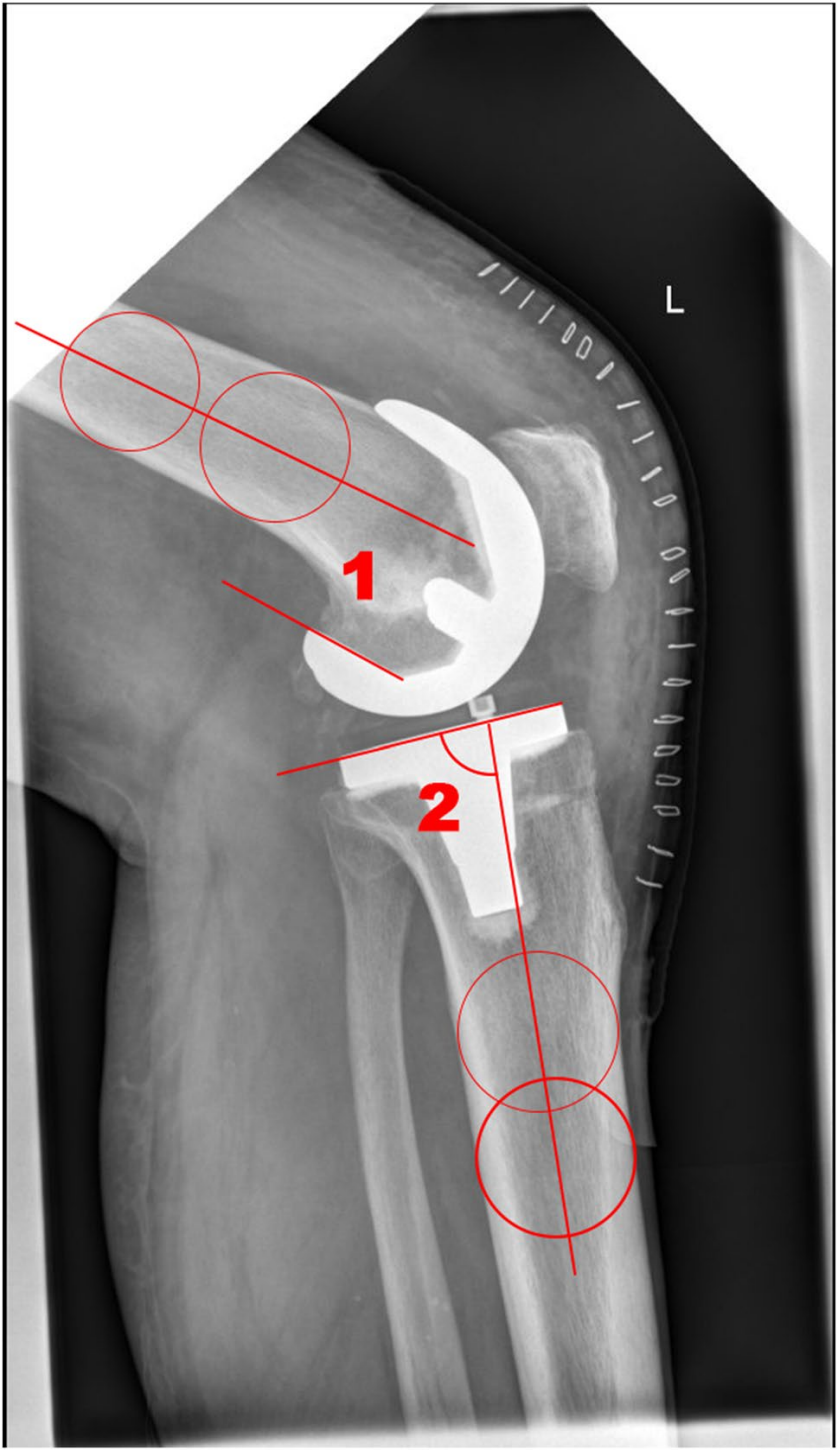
Measured angles on lateral view X-ray—femoral component flexion (1) and tibial slope (2)
